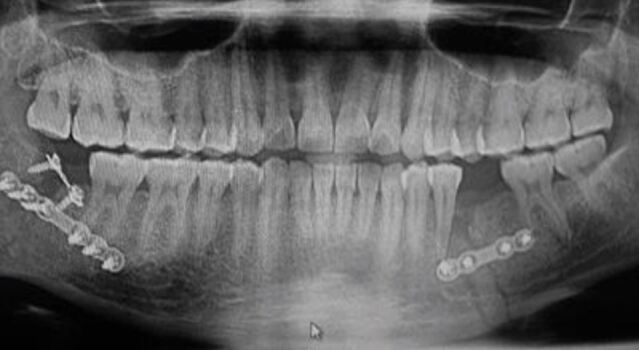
Radiograph of a fractured mandible following fixation
